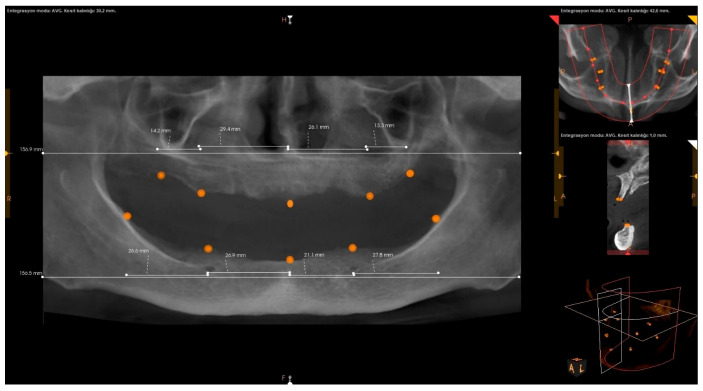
Landmarks on a panoramic radiograph.
